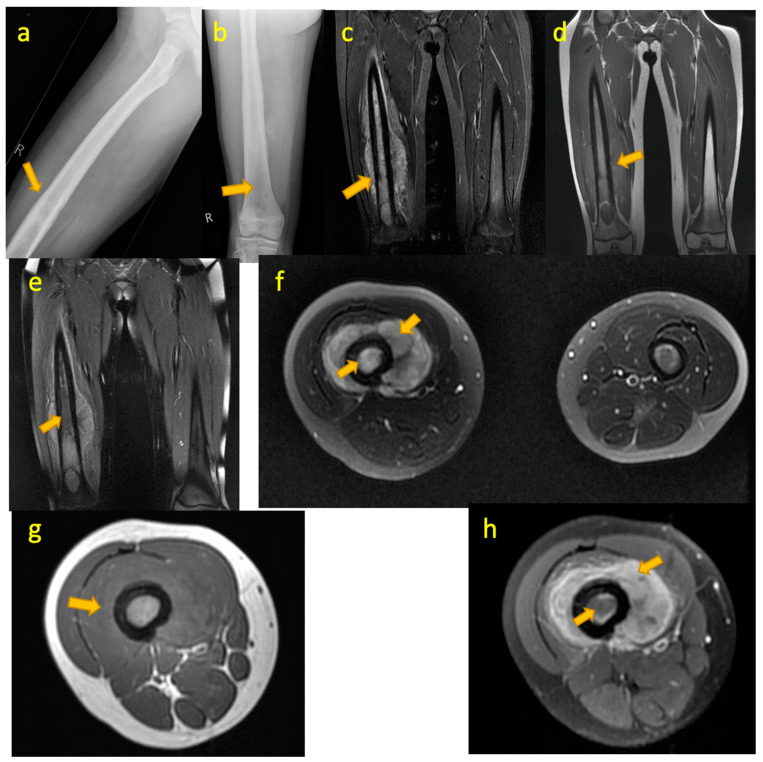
(a,b) Ewing sarcoma of the right femur in a 13-year-old girl who presented with a painful limp. X-ray of right femur lateral and AP views showing an ill-defined aggressive/permeative bone lesion in the mid and distal femoral shaft with periostitis and soft tissue mineralisation. (c–h) Coronal and axial STIR, T1W, and post-Gad T1W fat-sat MRI images reveal the extent of the bone lesion and associated large soft tissue component.
